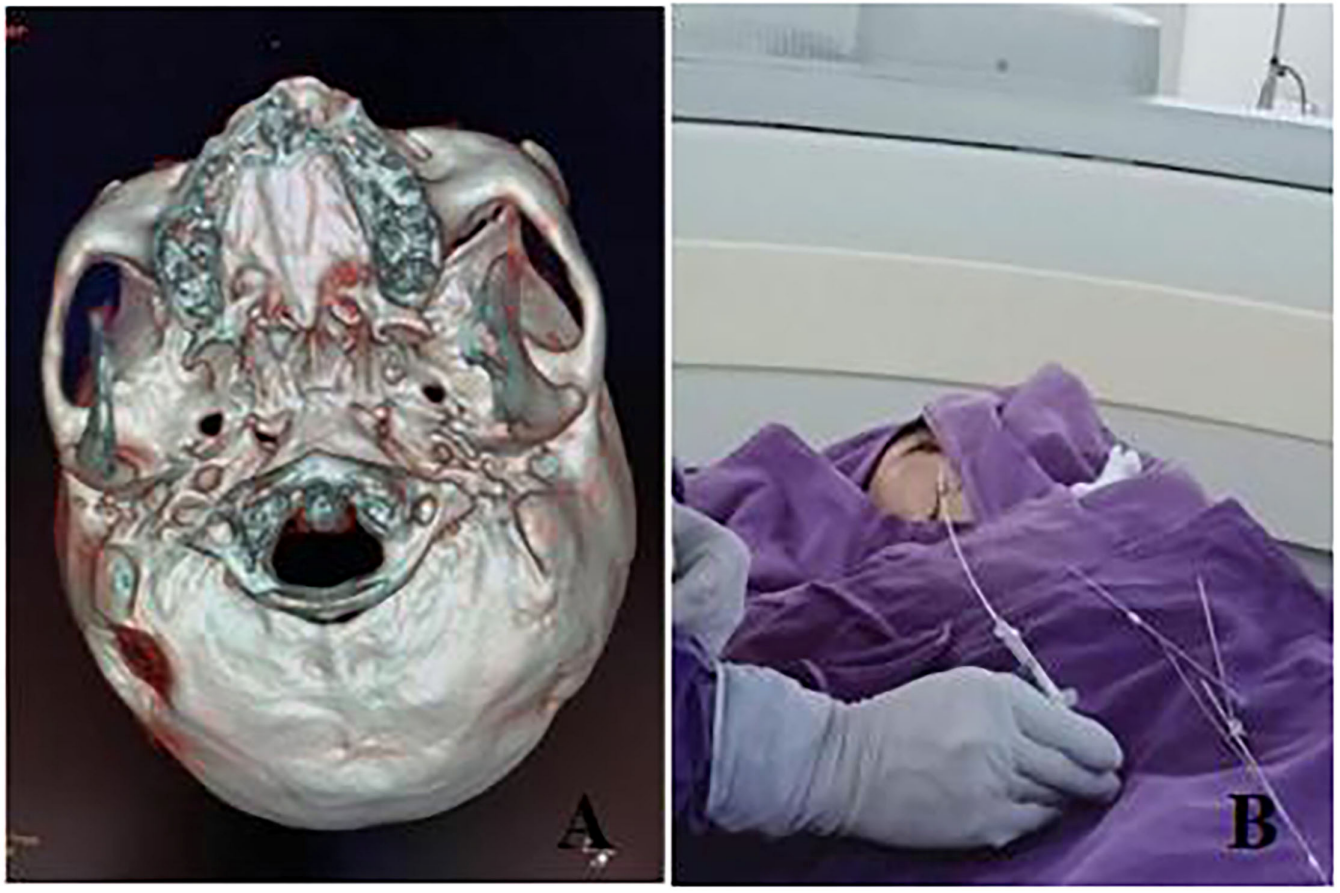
(A) 64-slice CT skull reconstruction was performed before surgery to check the direction and size of the foramen ovale; (B) Determine the direction of the patient's puncture.
